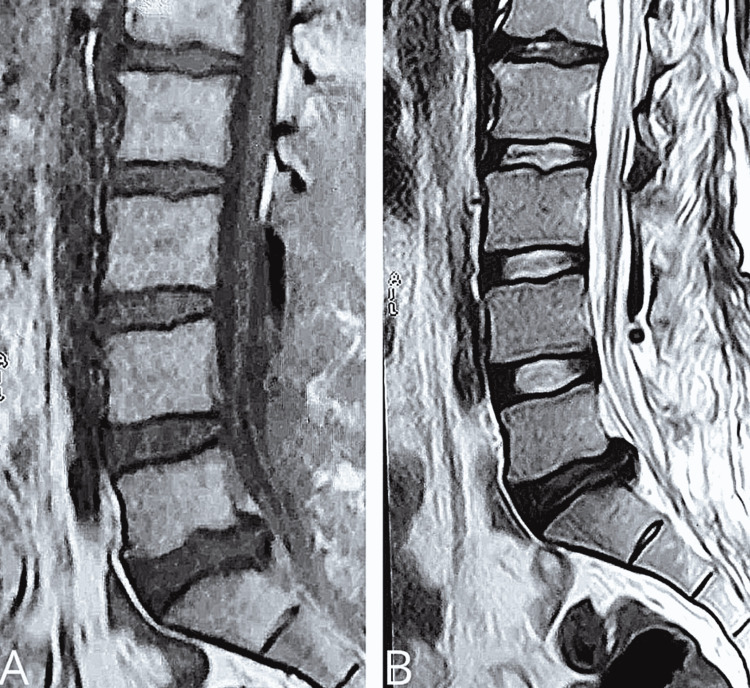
(A) T1 MRI and (B) T2 MRI images showing removal of the lesion.
